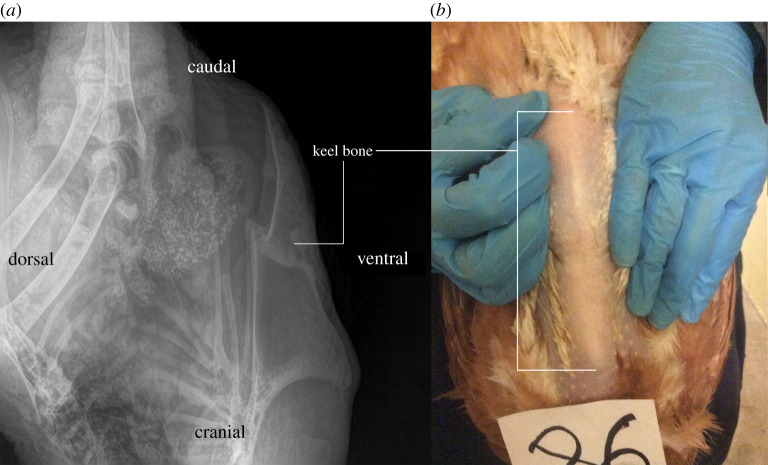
Images of an adult laying hen with a fractured keel bone. (*a*) a radiograph of a lateral view of the keel bone labelled with anatomical planes and, (*b*) a photograph of a frontal view of the keel bone and the surrounding skin, photo includes ID number.
